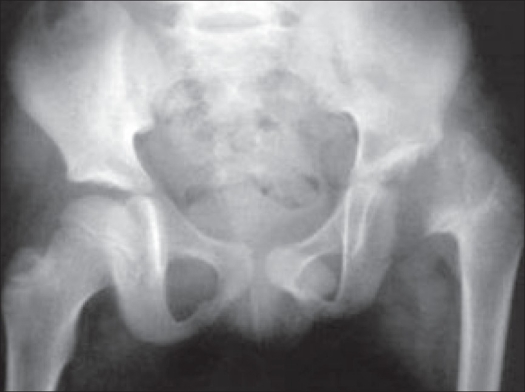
X-ray of the pelvis showing coxa breva with subluxed remnant of head of femur with deformed and bifurcated neck
